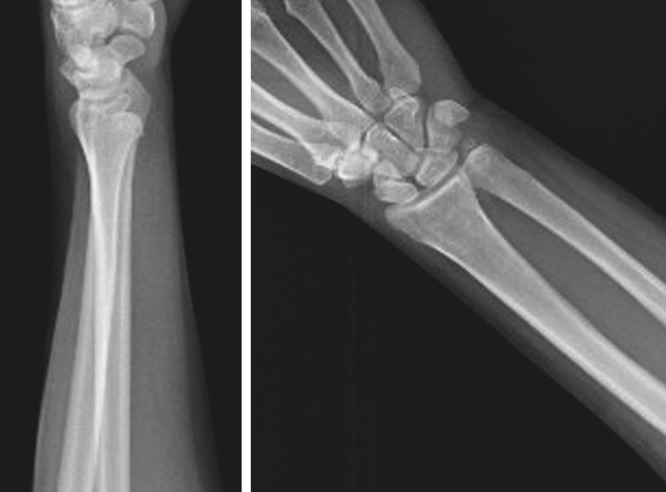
X-ray examinations. Antero-posterior and lateral views show the volar displacement of the ulna in relation to the DRUJ, even if the later view does not clearly show the dislocation, explaining the high index of suspicion necessary in this pathology.
